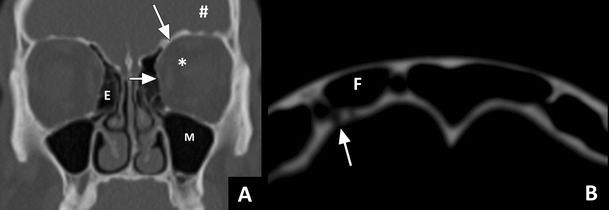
Sinus anatomy: a) Coronal CT image in bone window showing the right ethmoid sinus (E); the left maxillary sinus (M); the lamina papyracea (short white arrow); the left orbit (*); the orbital plate (long white arrow); the anterior cranial fossa (#). b) Axial image (WL 1500, WW 2600) showing very small congenital dehiscences (arrow) between the frontal sinus (F) and the anterior cranial fossa
